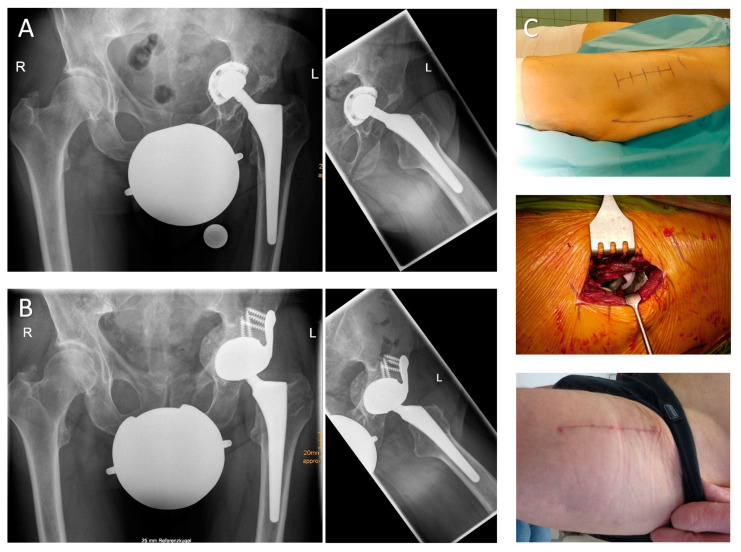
(A) A.p. and axial radiograph of a 66-year-old male patient with significant medial cup protrusion. The intraoperative defect classification revealed an intact anterior and posterior column with supportive rim elements (Paprosky type IIC). (B) The defect was filled with impacted allogeneic bone chips obtained from two femoral heads. A.p. and axial radiographs 12 months postoperatively demonstrate sufficient integration and remodeling of the graft and reconstruction of the anatomical center of rotation. (C) Pre-, intra-, and postoperative views showing the skin incision used for DAA revision arthroplasty and its position relative to the previously used approach (HHS: 66 preoperatively, 100 postoperatively; OT 149 min).
